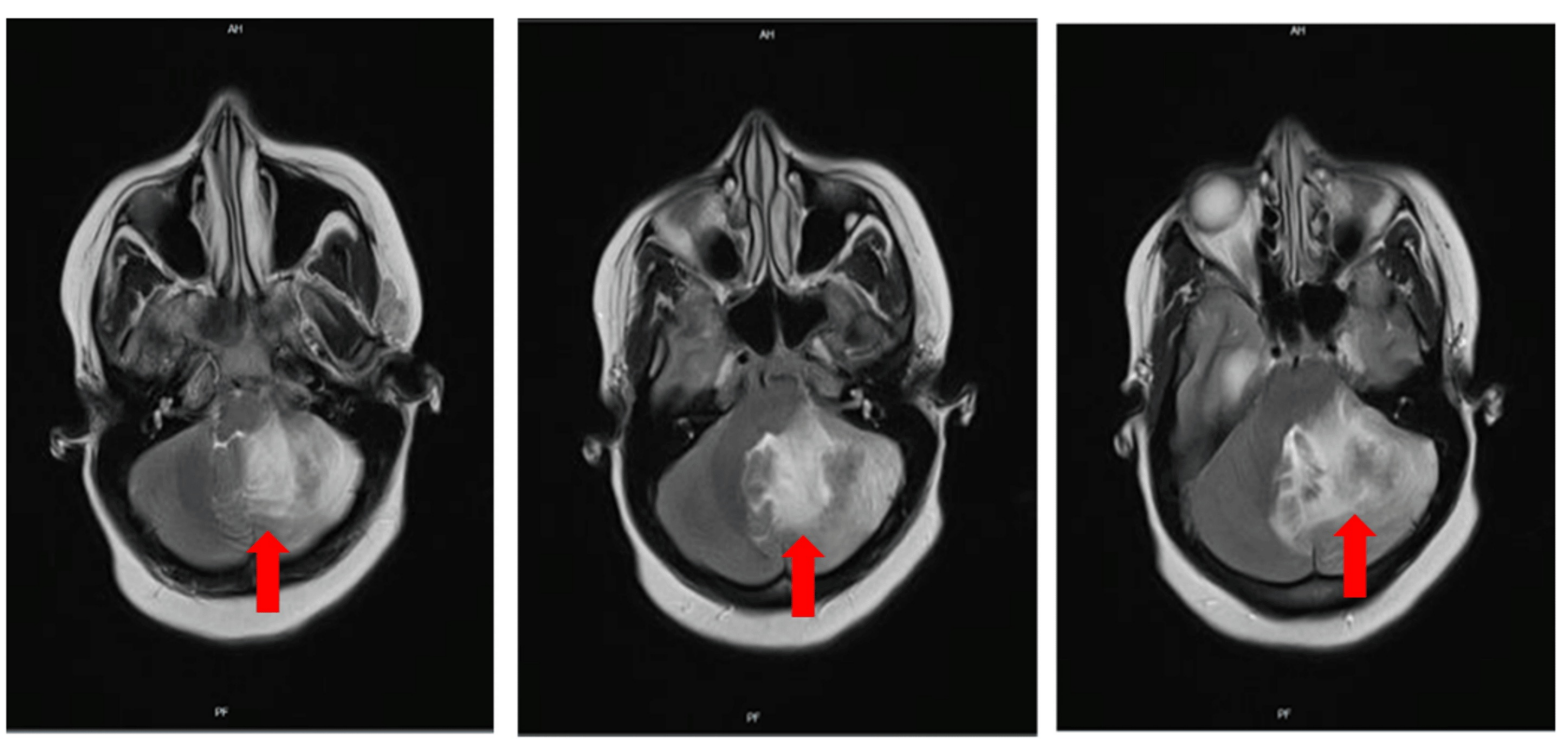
T1-weighted MRI of the brain in axial projection The red arrow marks the left cerebellar hemisphere lesion surrounded by severe perifocal edema causing mass effect and dilatation of the ventricular system.
